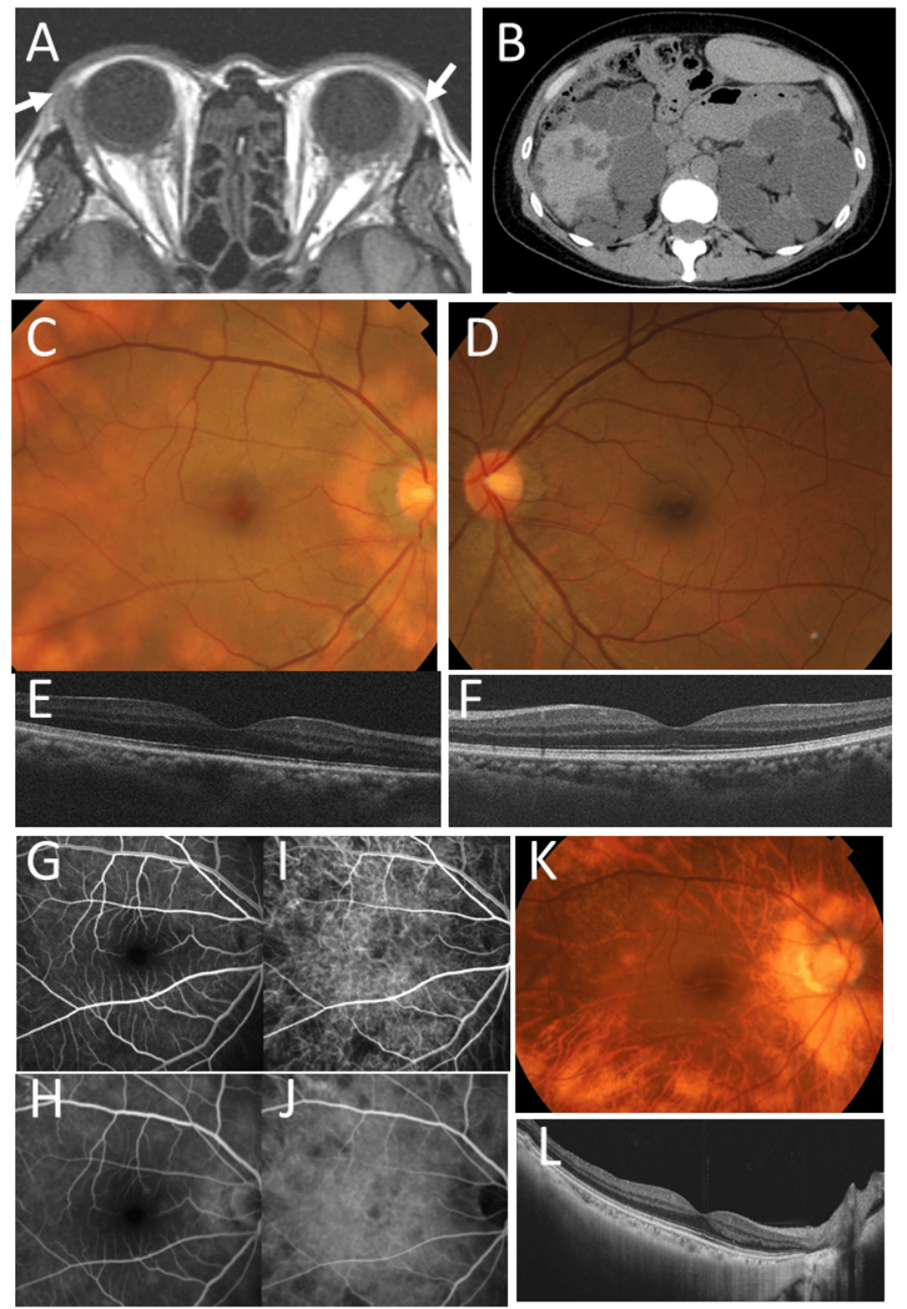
MRI, CT scan, and fundus images The MRI shows bilateral lacrimal gland enlargement at the age of 45 years (arrows; A). The CT scan shows bilateral polycystic kidneys at the age of 53 years (B). At the age of 49 years, fundus photographs (C) show yellowish spotty deep retinal to choroidal lesions in the right eye and normal fundus in the left eye (D). The optical coherence tomography in the horizontal section appears normal in the right eye (E) and left eye (F). The fundus angiography in the right eye shows early-phase no filling (G) and late leakage (H) with fluorescein dye and both early-phase (I) and late-phase (J) no filling with indocyanine green. At the age of 57 years, the fundus photograph shows mild degenerative retinochoroidal changes (K), while the optical coherence tomography reveals mild thinning of the choroid (L) in the right eye.
